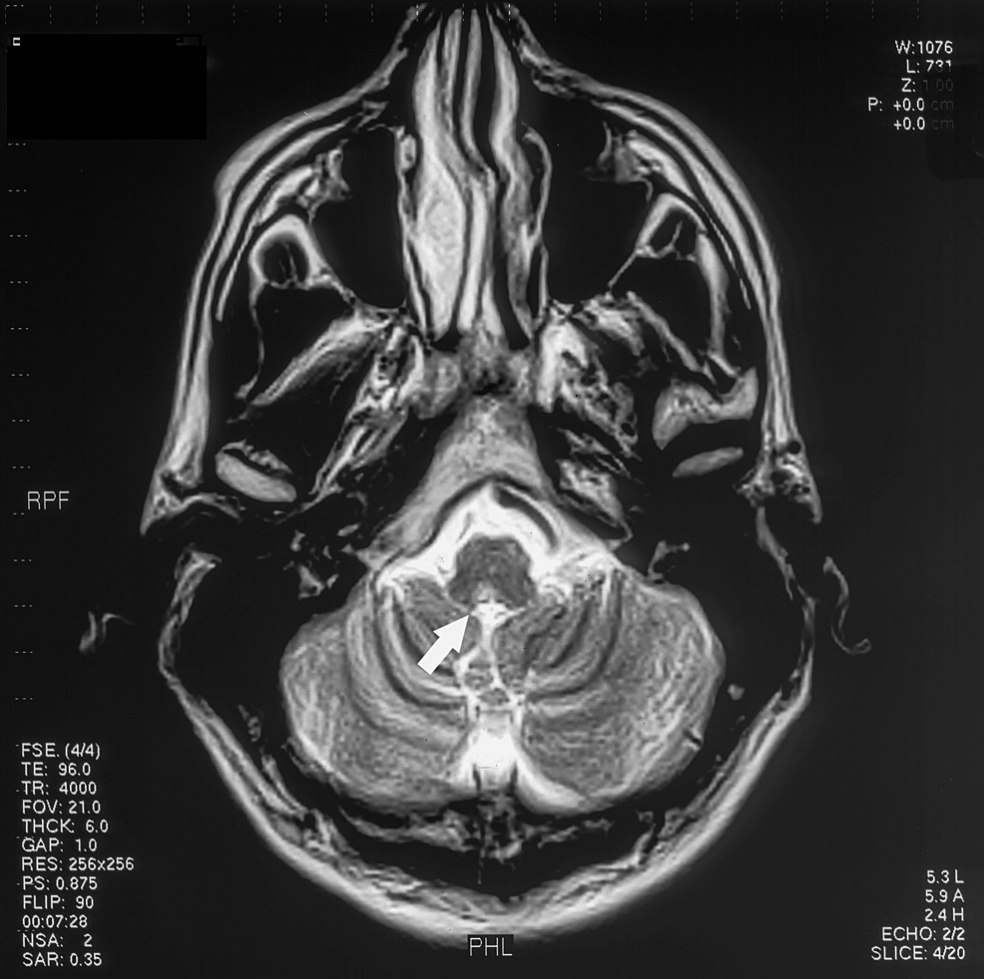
1T MRI axial Image showing an area of demyelination in the right medulla on the floor of the fourth ventricle (white arrow)
